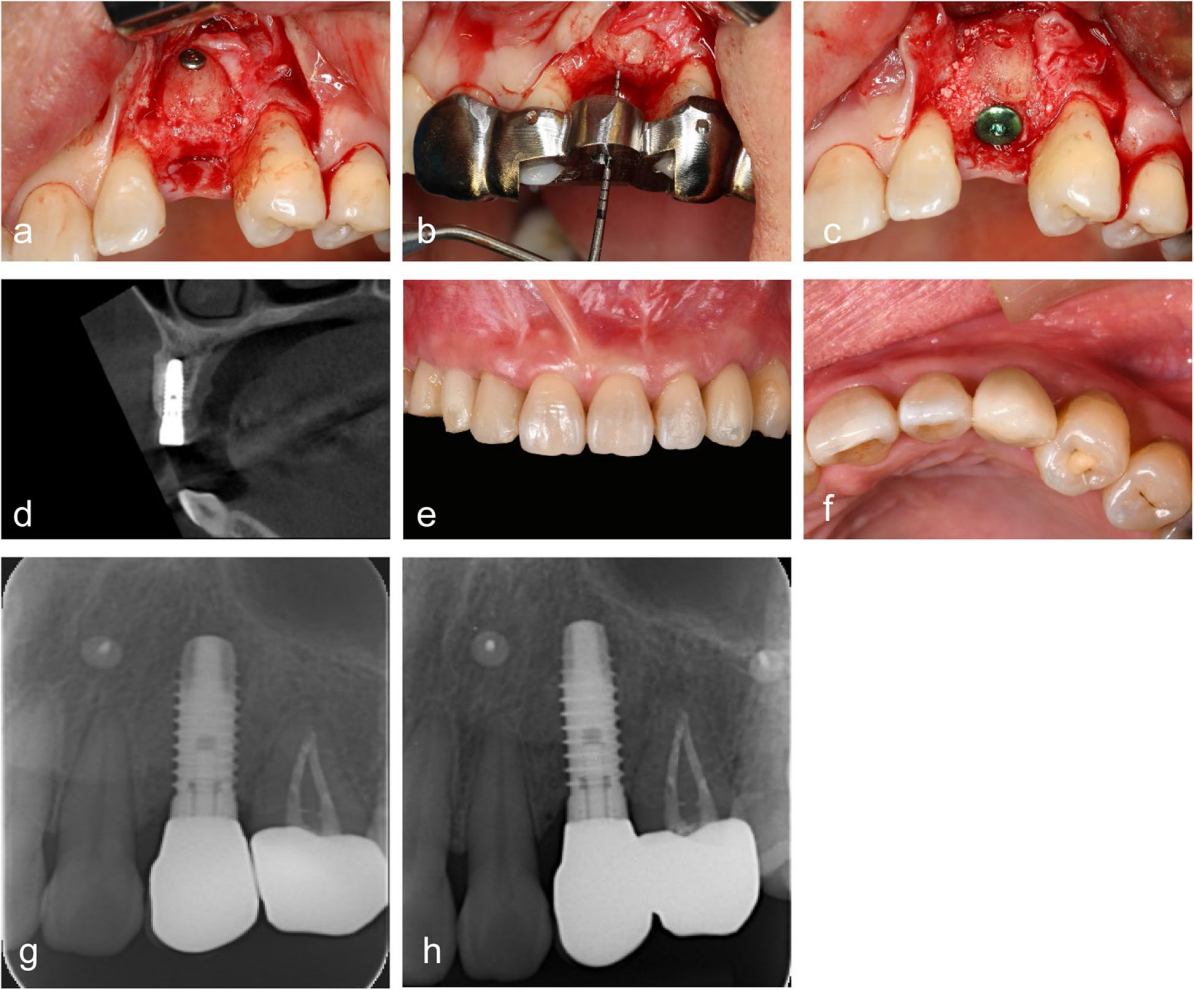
Implant placement and prosthesis loading. a Bone blocks with good osteogenic results after six months; b socket preparation following guidance of tooth-supported base template; c implant placement; d postoperative CBCT of bone block and implant; e–f screw-retained prosthesis; g X-ray of implant after final restoration; h X-ray of implant after 1 year of loading
